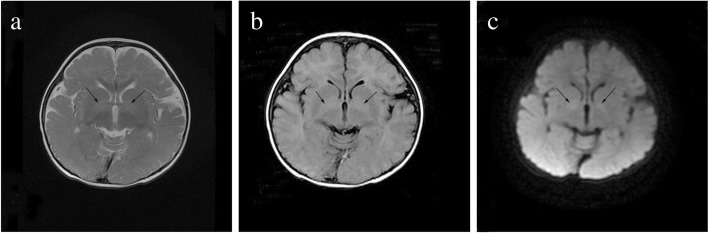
Cerebral MRI of patient 4 displayed hyperintense signal of bilateral globuspallidus (arrow), widened ventricle, and interval outside the cerebral on T2WI (a), FLAIR (b) and diffusion weighted image (c)
